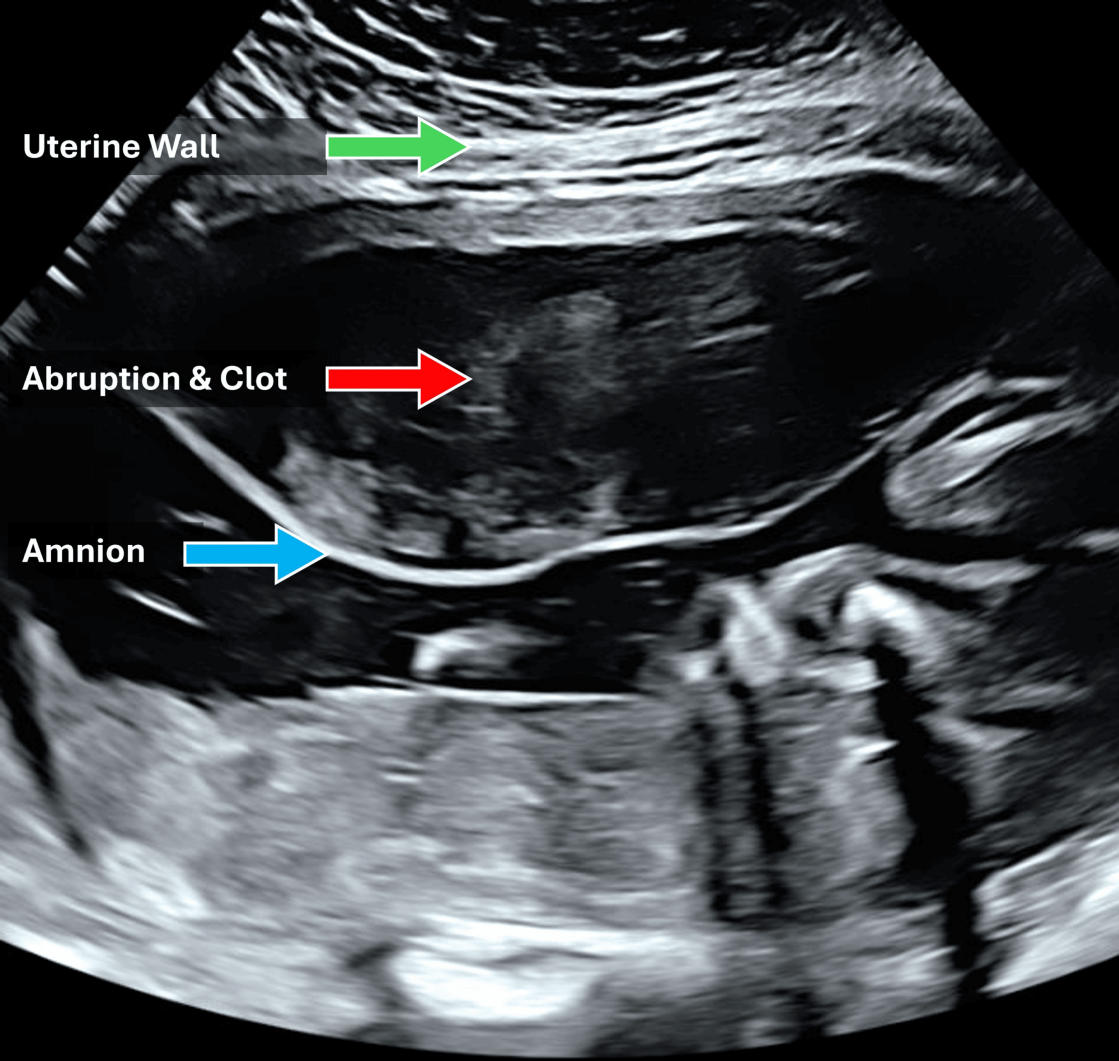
Transabdominal sonogram demonstrating acute placental abruption. An isoechoic layering appears to extend across the width of the sonogram field with a defined area of clot between the wall and amniotic sac.
